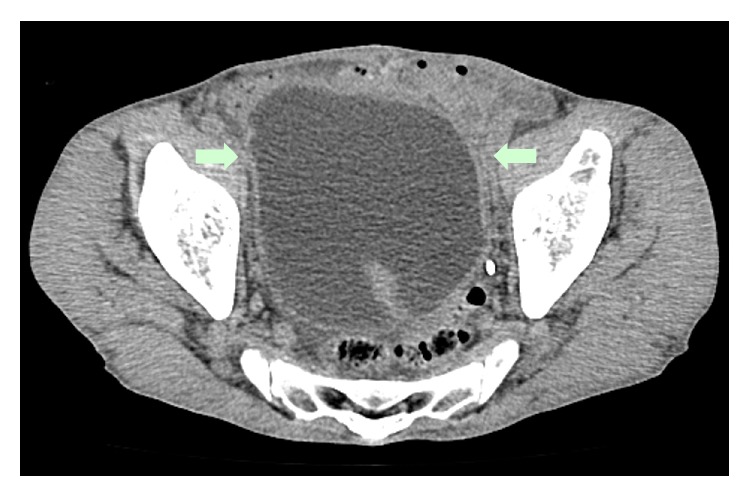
Compression of the bilateral common iliac veins (arrows) by the distended bladder on plain abdominal CT.
